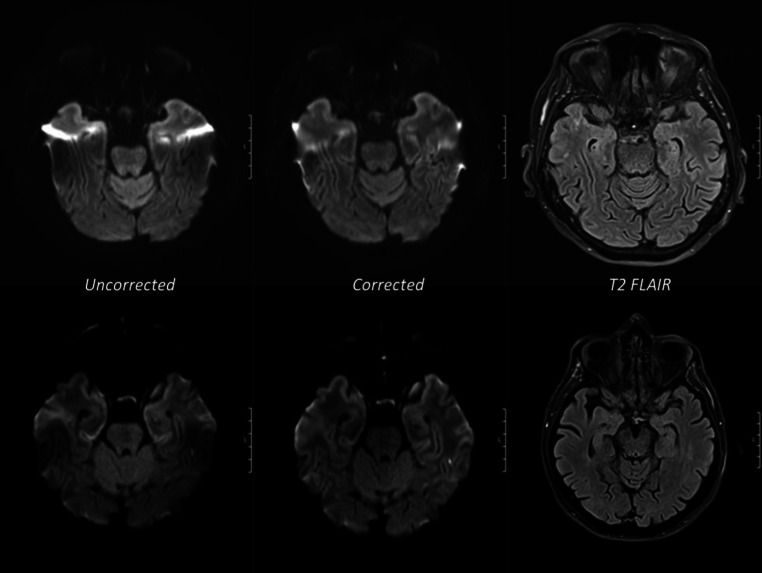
Examples of axial MRI scans of two different patients (*upper row*). Corrected EPI diffusion with a clear reduction in distortion artifacts affecting the temporal lobes. (*lower row*): corrected EPI diffusion showing improved conspicuousness of an ischemic lesion in the left temporal lobe. Matched T2 FLAIR images are provided for comparison
